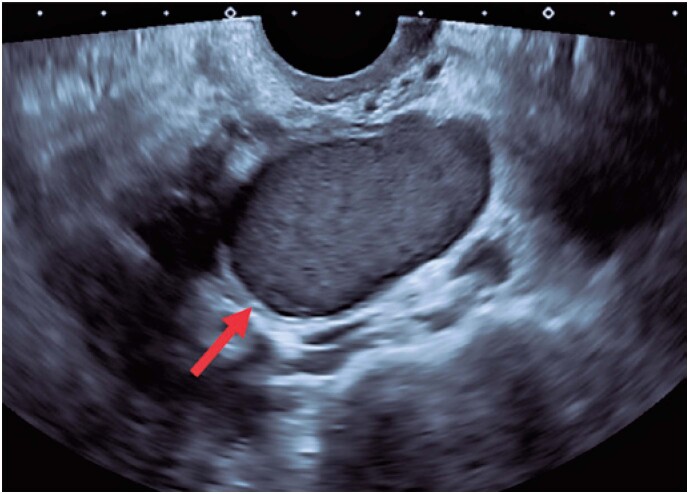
A sonographic transvaginal image of a cyst with homogeneous hypoechogenic content (“ground glass”) in the left ovary (arrow), which suggested endometrioma
